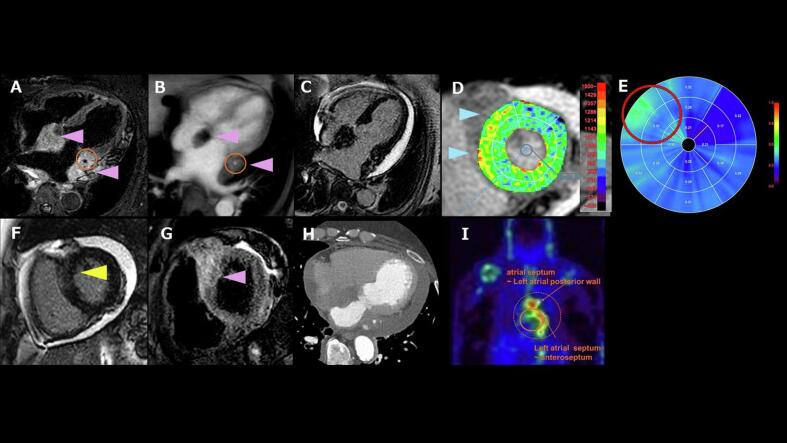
Cardiac Magnetic Resonance Imaging. (A) Black blood T2-short tau inversion recovery (T2-STIR) 4-chamber view. (B) Early enhancement of gadolinium. (C) Delayed enhancement of gadolinium, 4-chamber view. (D) Native T1 mapping in basal left ventricle. (E) Extracellular volume for all segments in left ventricle. (F) Delayed enhancement of gadolinium of basal left ventricle. (G) T2-STIR of basal left ventricle (H) Gallium-67 scintigraphy. (I) Contrast enhanced cardiac computed tomography. T2-STIR (A and G) revealed high signal intensity in the interatrial septum and left atrial wall and basal anteroseptal region of interventricular septum (purple arrowheads). The structure of the coronary arteries was preserved (A and I, orange circle). Delayed enhancement of gadolinium (F) showed the basal areas in left ventricular wall (yellow arrowhead) which was consistent with high native T1 (D: blue arrowhead), high extracellular volume fraction (E: red circle), and high intensity on T2- STIR (G). Gallium-67 scintigraphy (H) found no evidence of other sites of malignancy and showed accumulation in the mediastinum and left ventricle, which suggested left ventricular infiltration.
